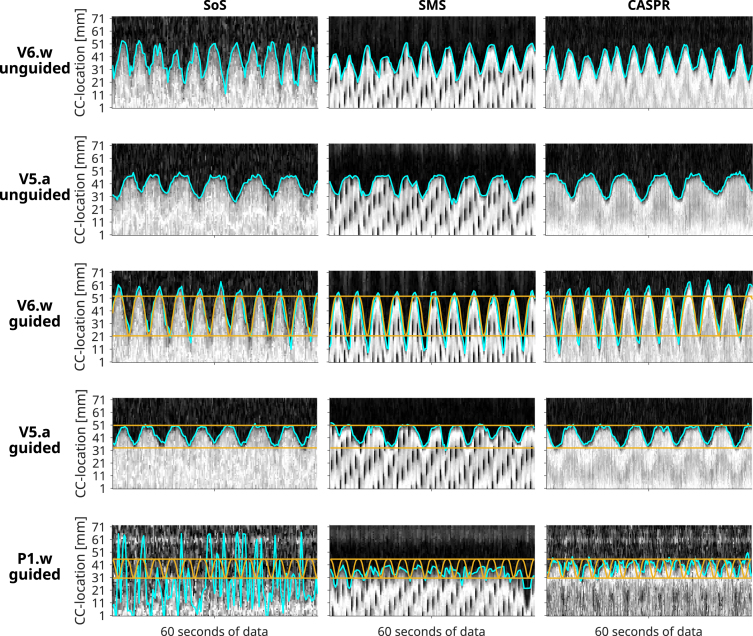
Examples of three subjects of the one-dimensional respiratory navigator data at the liver-lung interface (black is lung, white is liver) with the guidance in amber superimposed for the stack-of-stars (SoS), simultaneous multi-slice (SMS), and Cartesian acquisition with spiral ordering (CASPR) sequences. Extracted breathing waveforms are indicated in cyan. Guidance improved the breathing regularity. Note the signal saturation effects in the navigator data for the SMS sequence as a result of the axial image acquisition. For the patient (P1.w), no unguided 4D-MRI acquisitions were performed and the navigator during the SoS acquisition lacked quality required for guidance.
